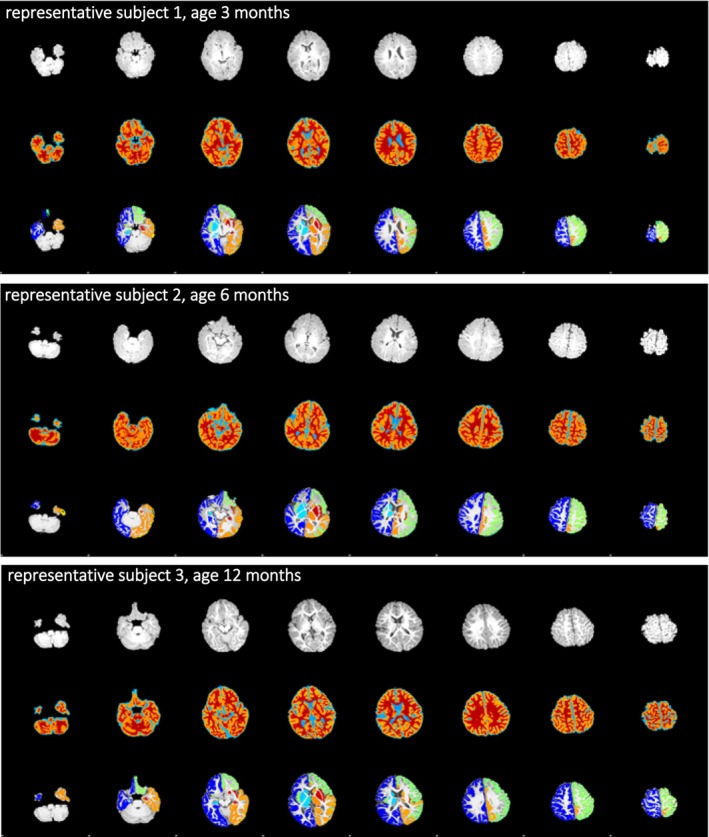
HyperDenseNet brain segmentations of three representative subjects with metopic synostosis (aged 3, 6 and 12 months, respectively). In each frame, the first row shows the plain axial MRI scan, the second row shows segmentation into grey matter, white matter and CSF and the third row shows segmentation of grey matter into subregions.
